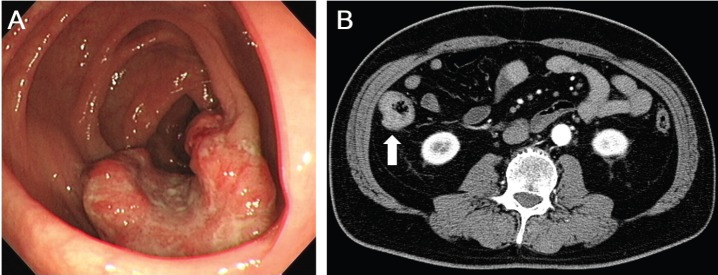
Images of the ascending colon with a cancerous lesion. (A) Colonoscopy examinations show an ulcerated tumour in the ascending colon. (B) CT scanning with contrast media reveals a mucosal thickness in the ascending colon without lymph node involvement or metastatic tumours.
